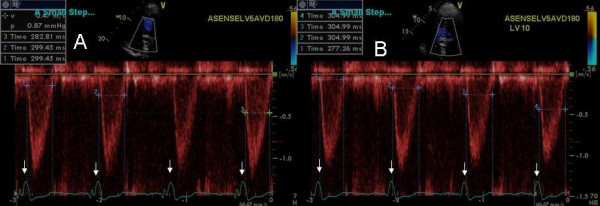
Left ventricular outflow tract pulsed wave Doppler velocity at LV pre-excitation of 5 ms (A) and LV pre-excitation of 10 ms (B). An increase in LV ejection time is shown in panel B. Also note the narrowing of QRS complex in panel B compared to panel A (white arrow heads).
